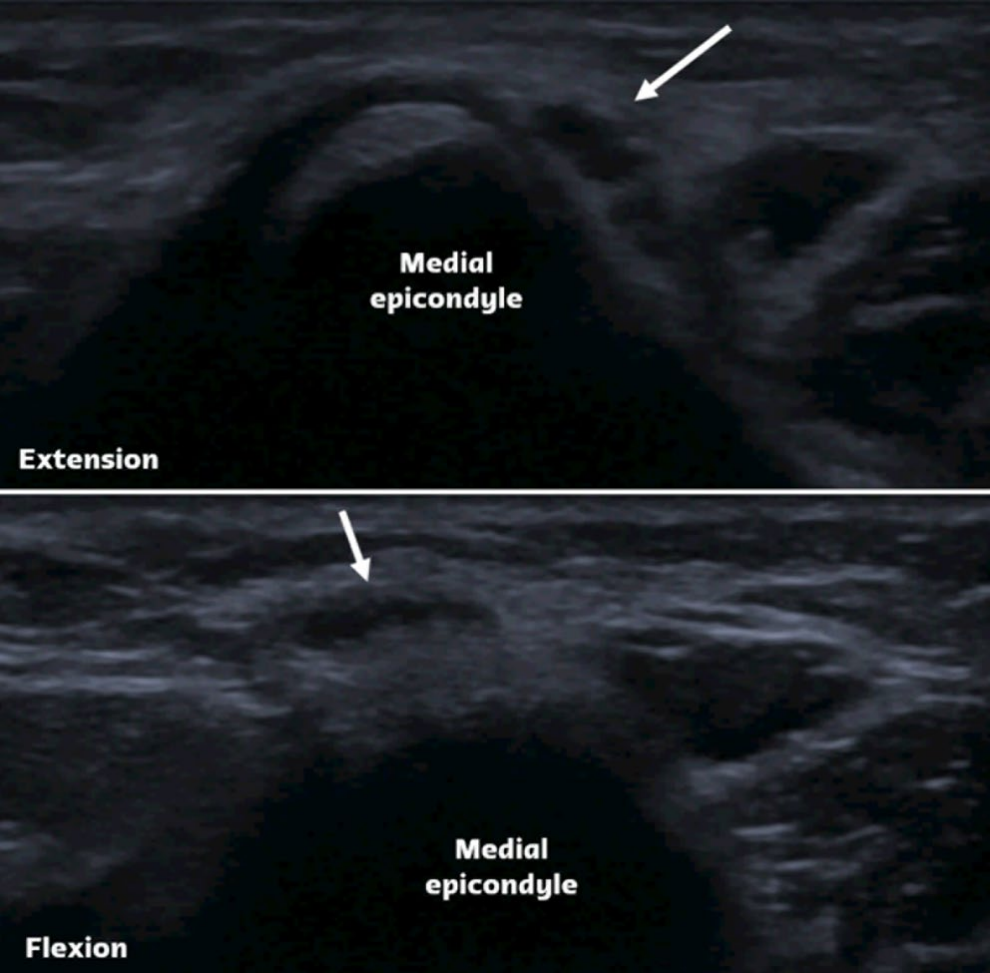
Ulnar nerve subluxation. Ultrasound images of the elbow in extension and flexion demonstrate the positional change of the ulnar nerve. In extension, the ulnar nerve is visualized posterior to the medial epicondyle (arrow). During flexion, anterior subluxation of the ulnar nerve occurs, with the nerve translocating beyond the apex of the medial epicondyle (arrow)
